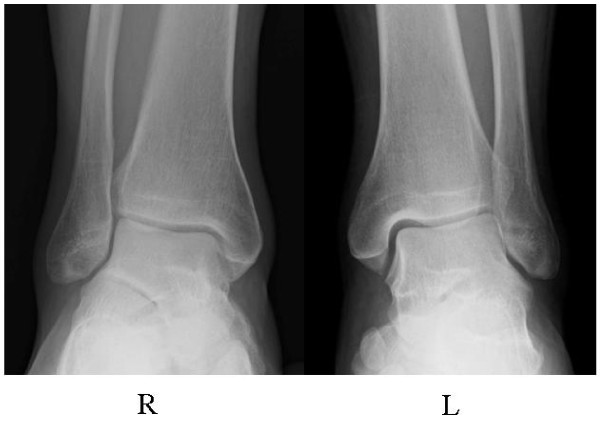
Radiographs of the both ankles after treatment. The regional osteoporotic change in the right ankle (Figure 1A) completely returned to normal, and findings were comparable to those of the left ankle at 32 months after the start of bisphosphonate treatment.
